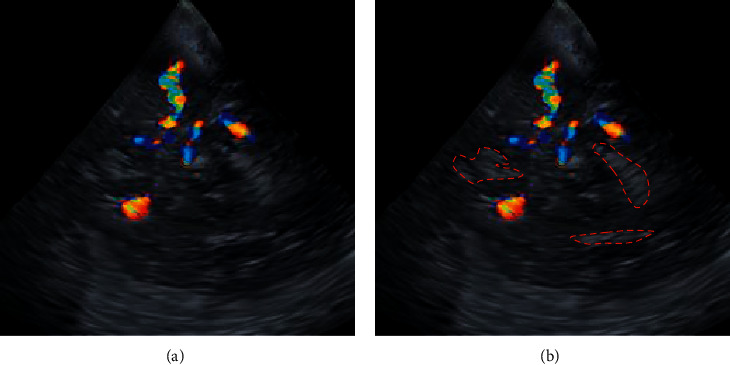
Repair of fetal ultrasound MAC blood flow images by differential equation models.
